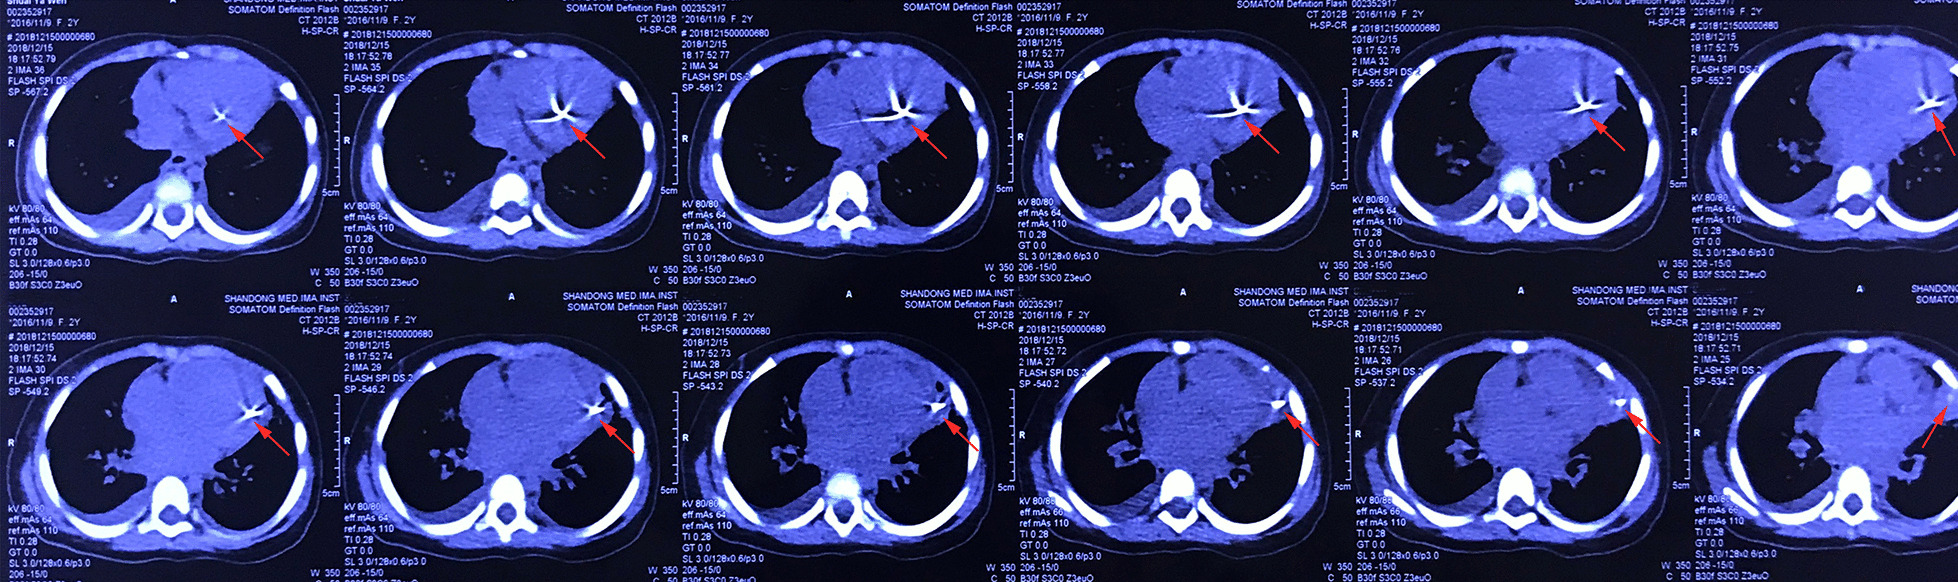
High-quality thoracic CT. Arrows indicated the entry, exit and extension of the needle through the heart
